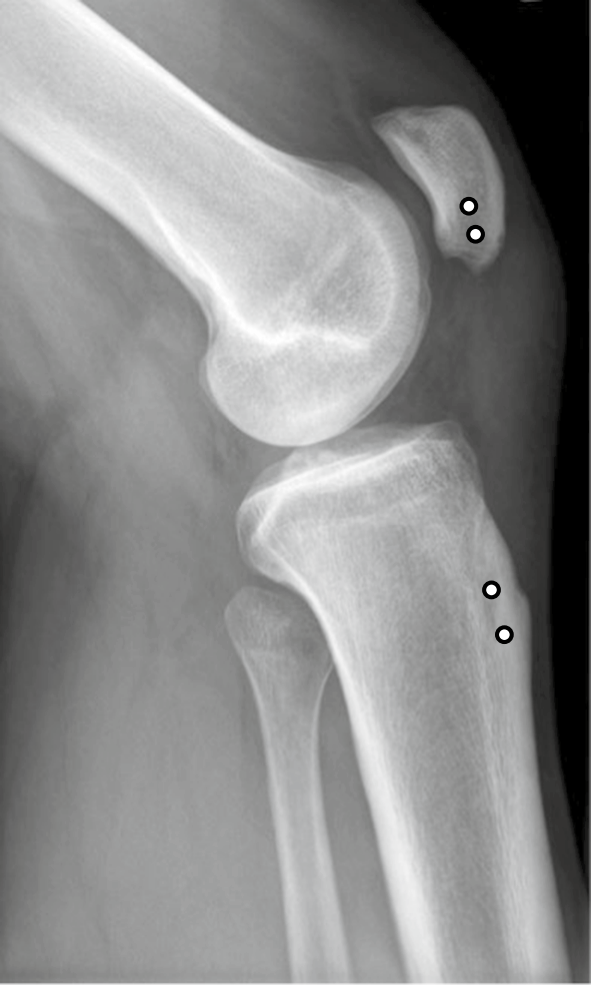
Preoperative lateral radiograph showing a patella alta in a patient with a patellar tendon rupture. The recommended tunnel position for the suture augmentation is marked (white circle with black border)
